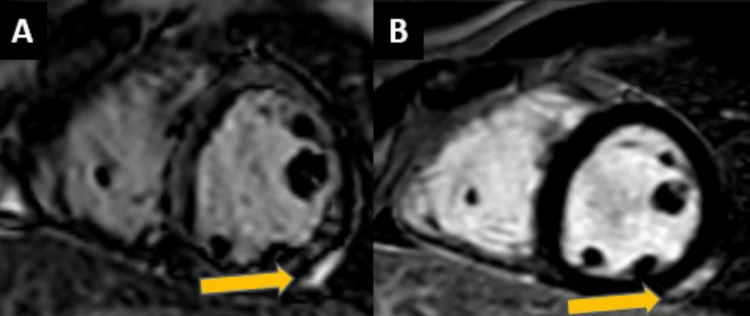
Gadolinium-enhanced MRI showing enhancement in the myocardium and adjacent pericardium.
